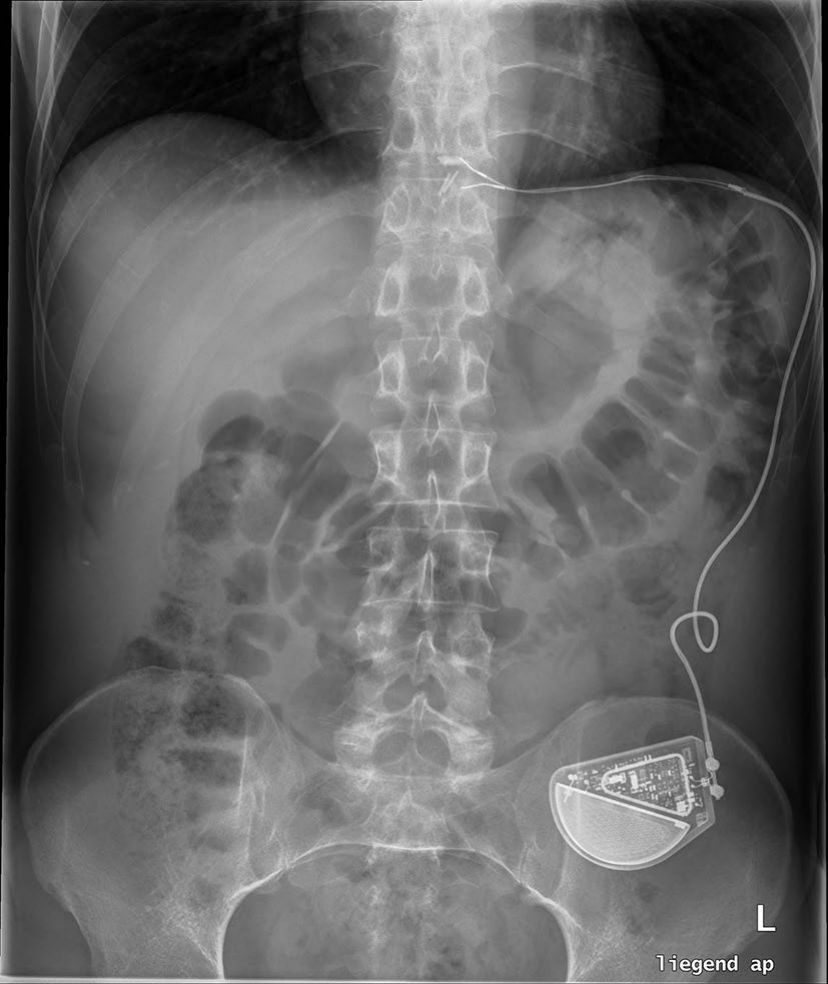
Abdominal X-ray showing the position of the electrodes and the implantable impulse generator (IPG)
